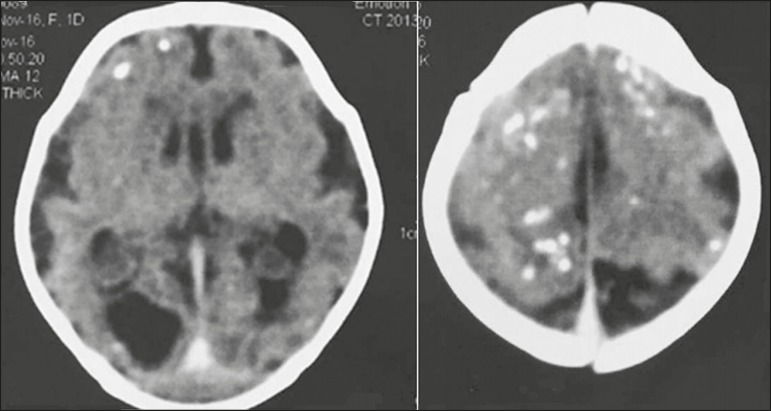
Non-contrast-enhanced computed tomography of the brain in a one-day-old
